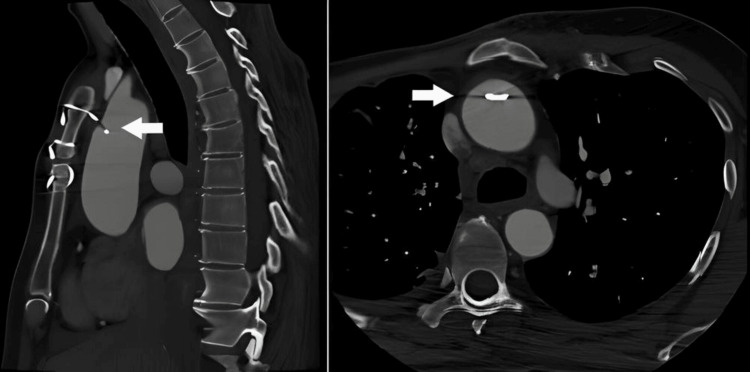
Computed tomography angiography showing a sternal wire penetrating the ascending thoracic aorta
